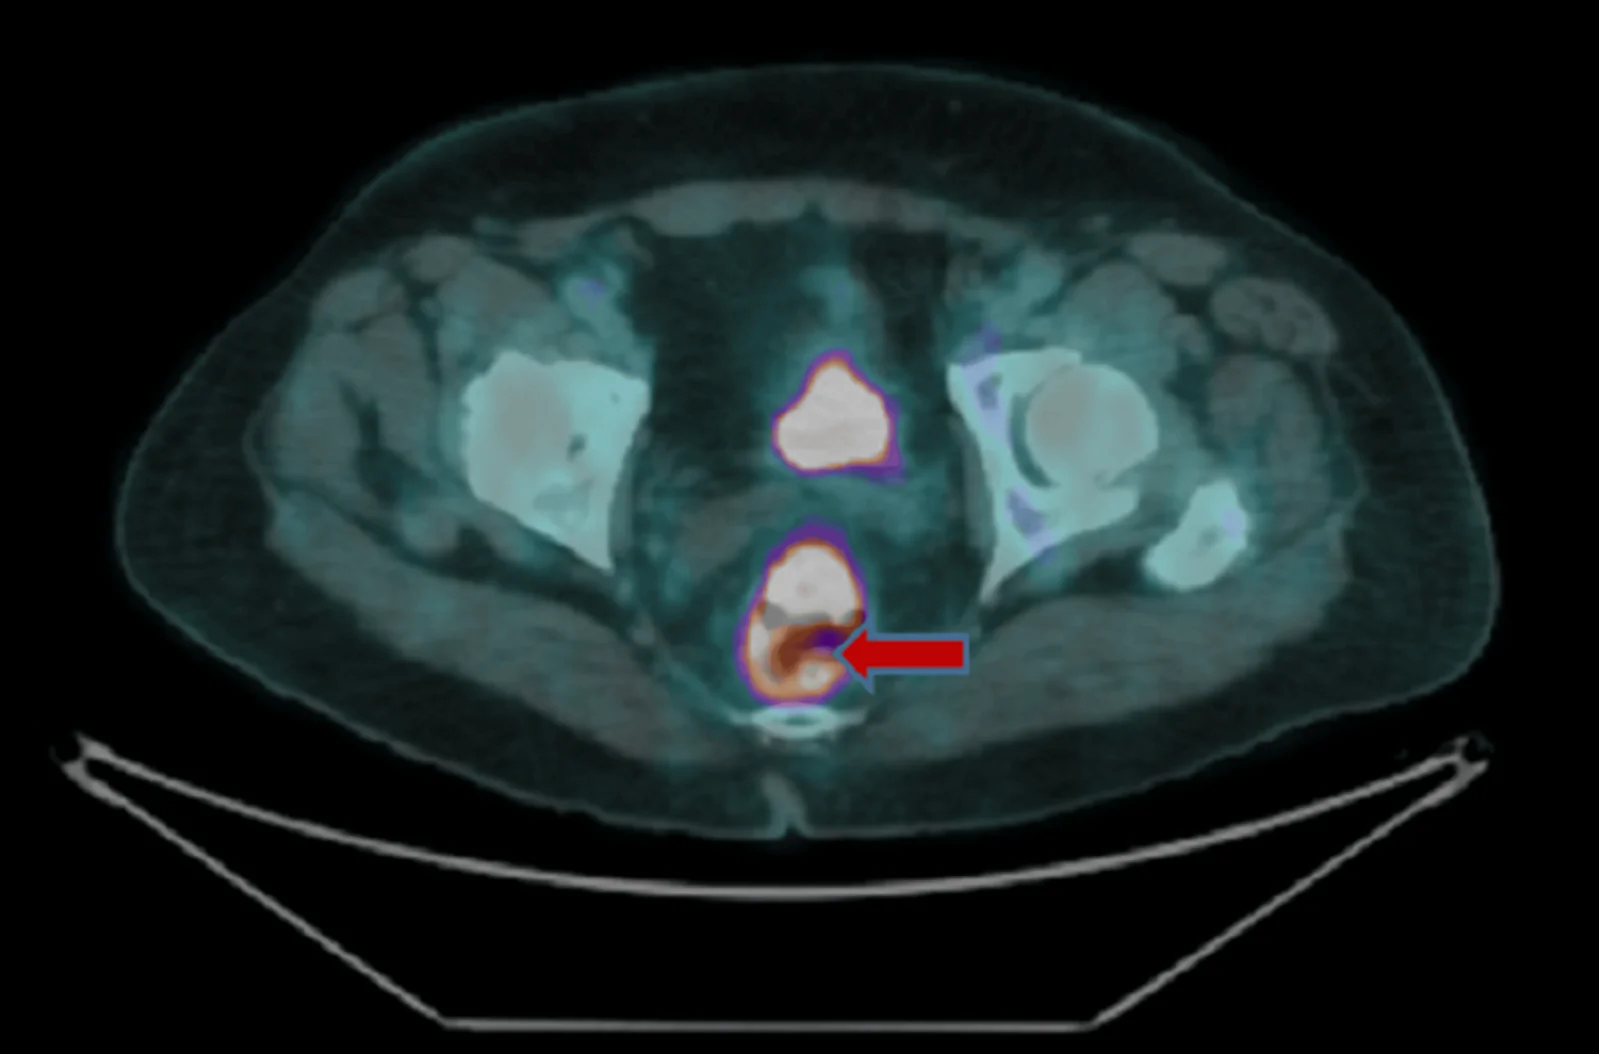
Positron emission tomography computed tomography showing the rectal tumor before treatment (arrow)
